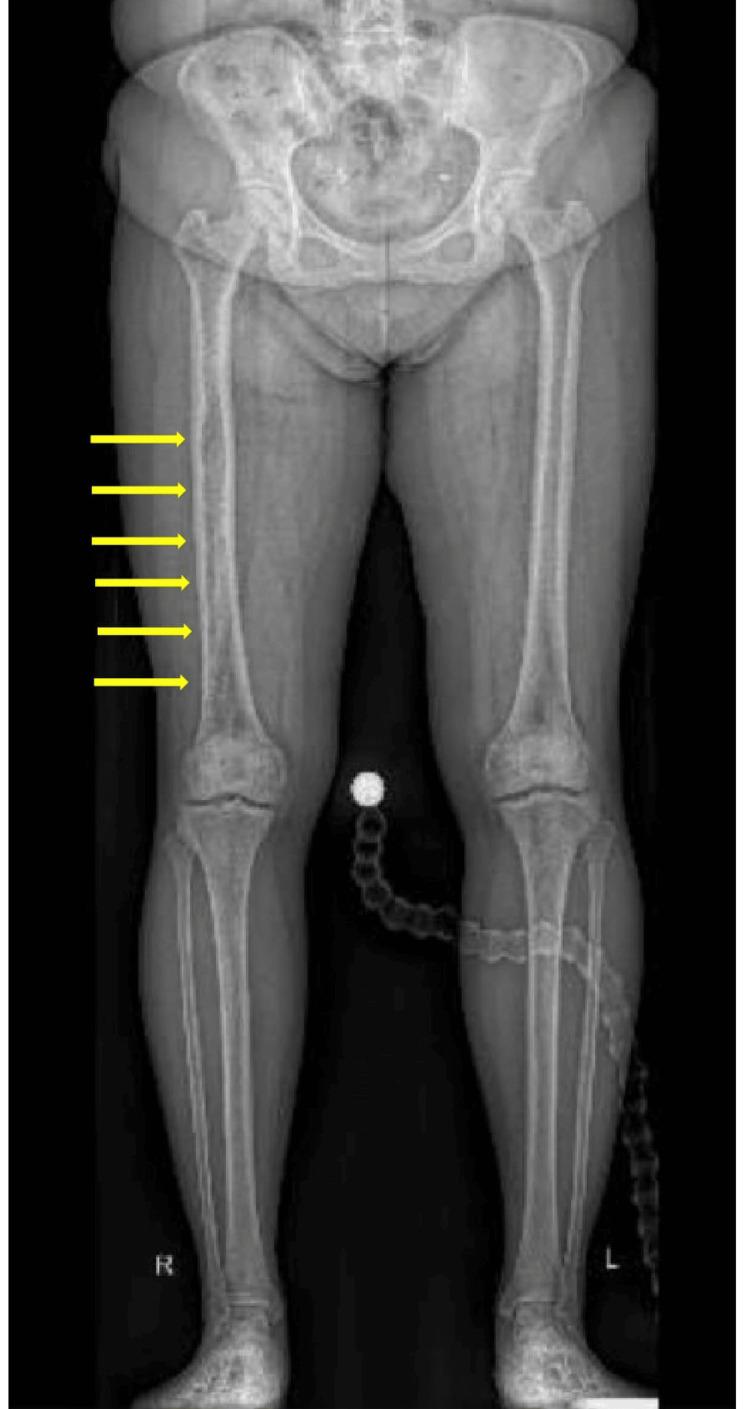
The lower limb plain radiograph delineated an ill-defined lucent intramedullary lesion in the right distal femur, extending to the proximal one-third of the femur (yellow arrows).
